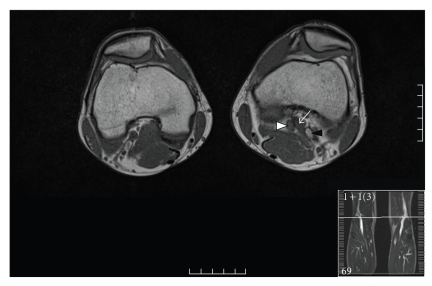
Axial T1-weighted MRI images through both knees at the level of the popliteal fossa showing the medial head of the gastrocnemius muscle (white arrow) going between the popliteal artery (white arrow head) and vein (black arrow head).
